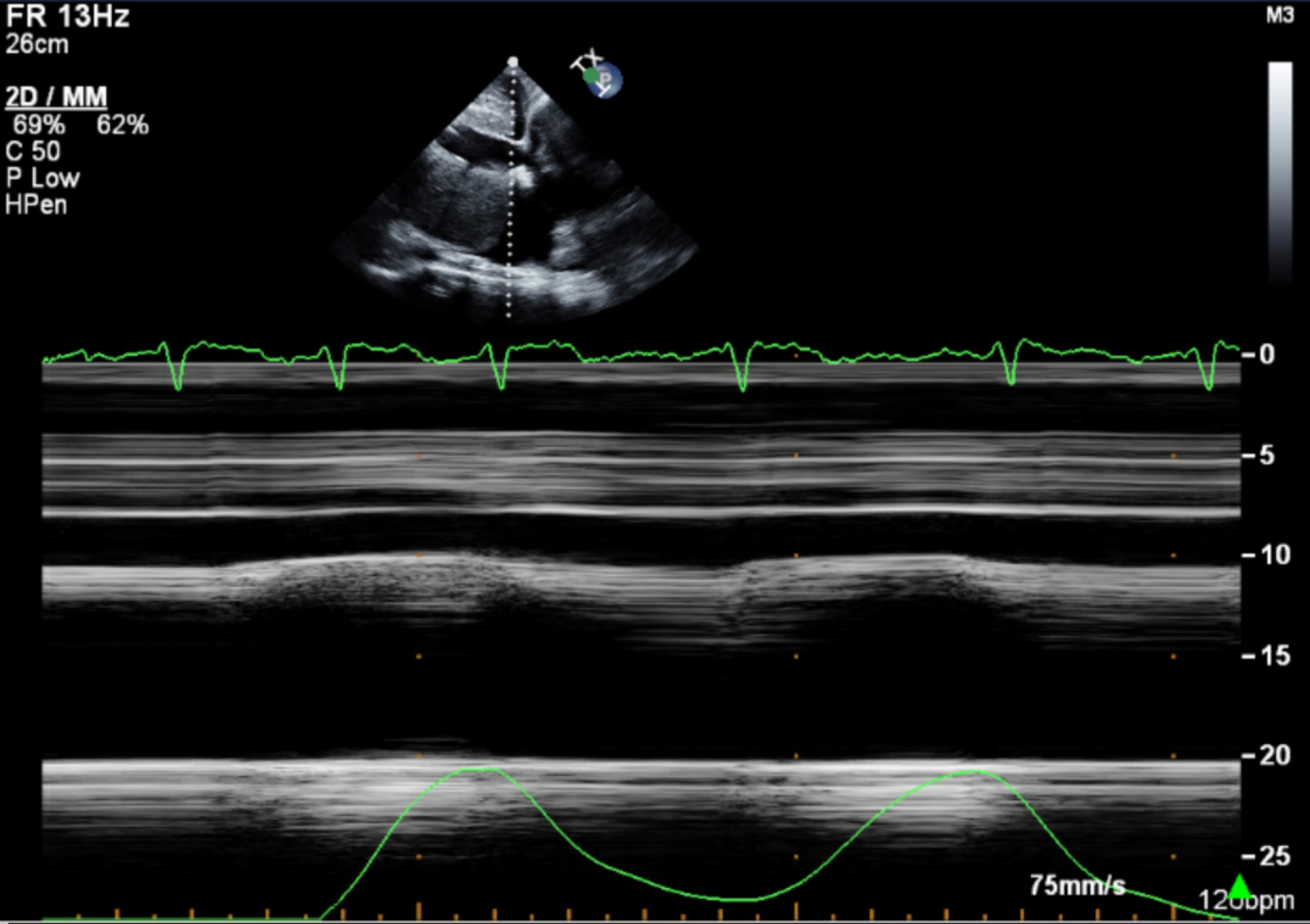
M-mode on transthoracic echocardiogram at admission showing non-compressibility and blunted respirophasic motion of inferior vena cava
